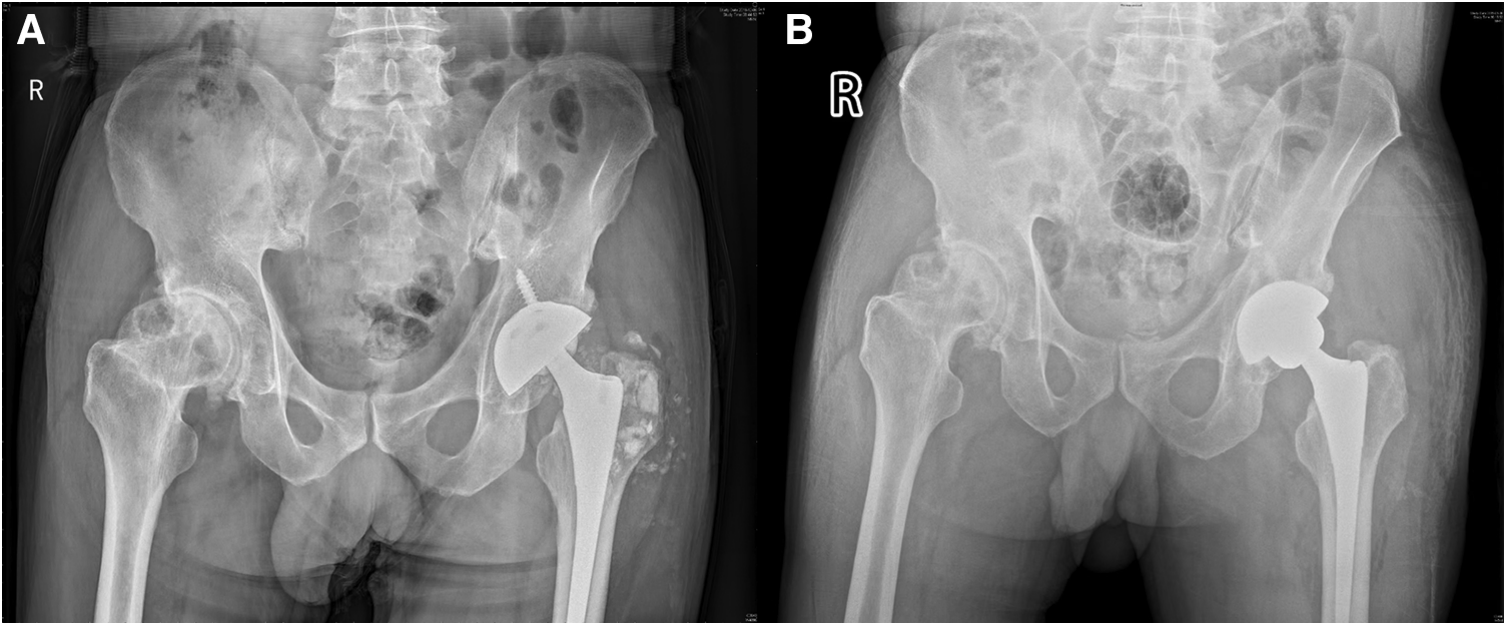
Pelvic and hip x-rays in case 2. (A) Preoperative anteroposterior radiographs of both hips. (B) Postoperative bedside DR anteroposterior radiographs of both hips.
